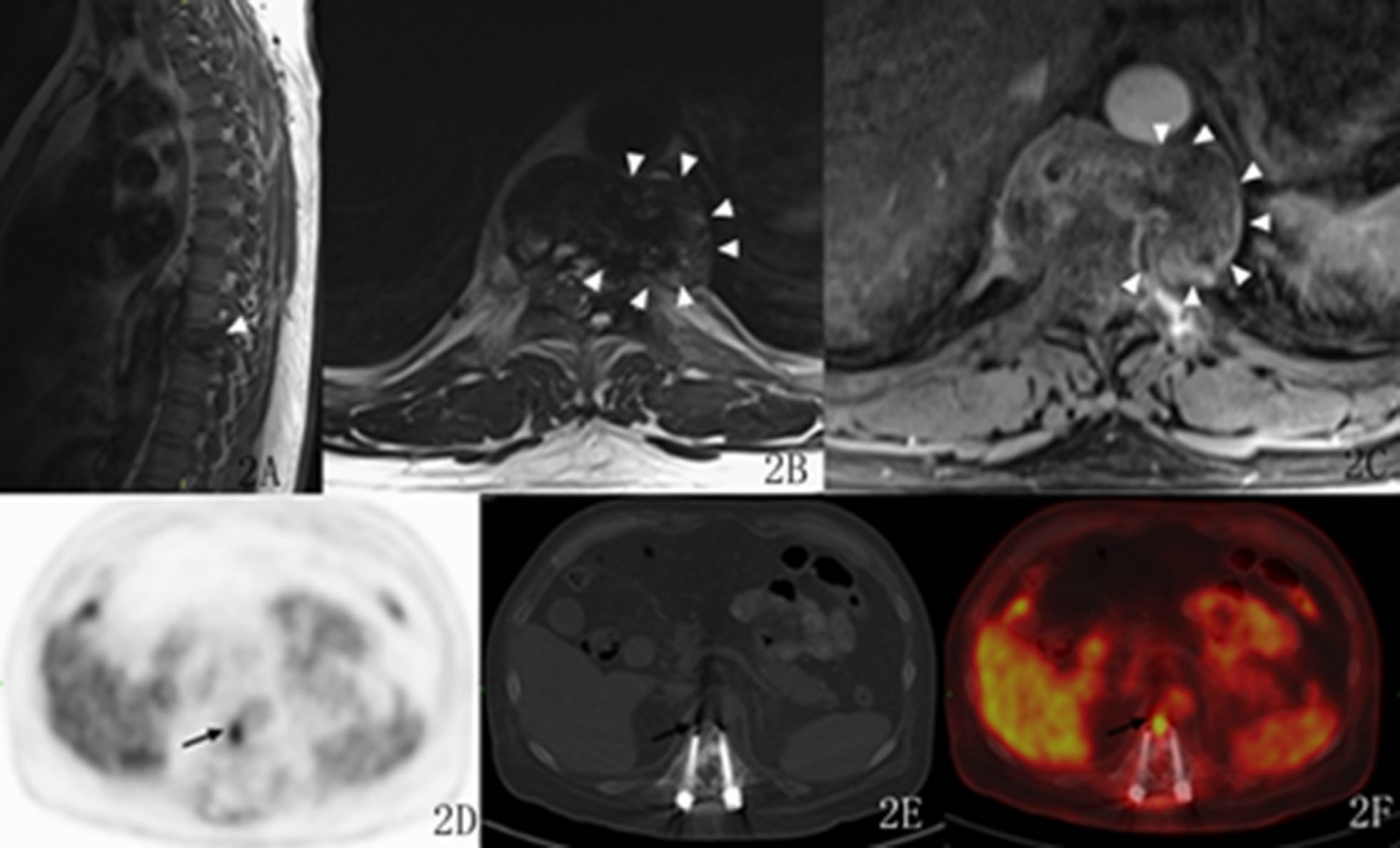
A 45-year-old male with benign GCTTS. The lesion was hypointense on T1WI (A, arrow) and mostly hypointense on T2WI in the T9 vertebra (B, arrows). Enhanced MRI scans revealed heterogeneous enhancement (C, arrows). Recurrence was suspected after surgery, and PET showed high ^18^F-FDG uptake in the vertebral body (D, arrow). The lesion was blurred on CT because of the interference from artefacts (E, arrow), but the fusion image clearly showed the high ^18^F-FDG-uptake lesion at the anterior edge of the T11 vertebra (F arrow)
